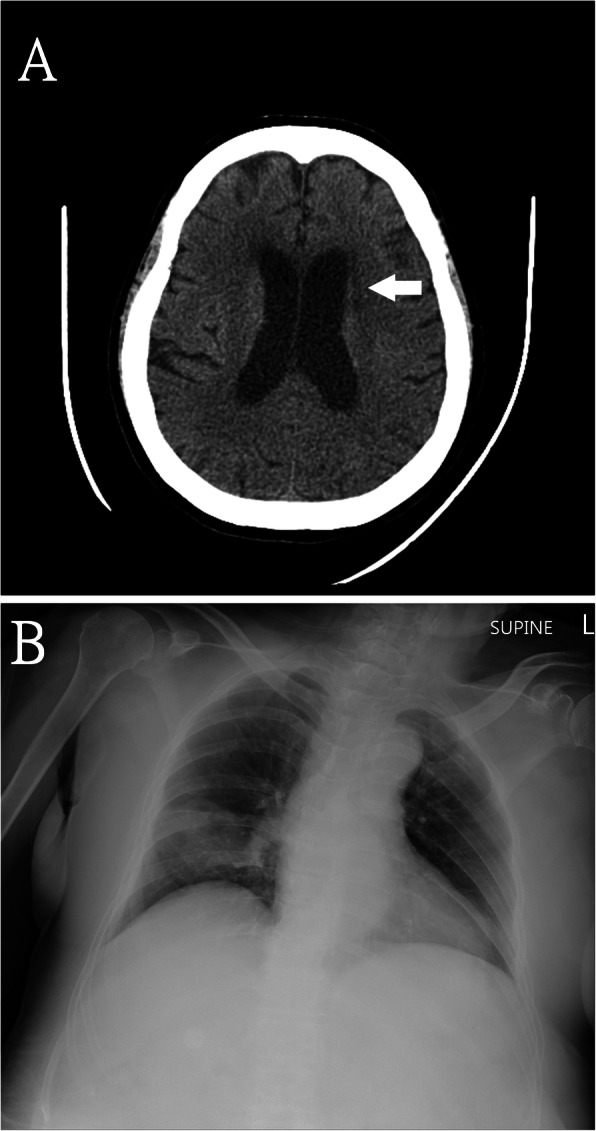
The brain computed tomography and chest radiograph. The brain computed tomography scan revealed a hypodense region in the left corona radiata (Fig. 1a). The chest radiograph revealed right middle lobe pneumonia (Fig. 1b)
